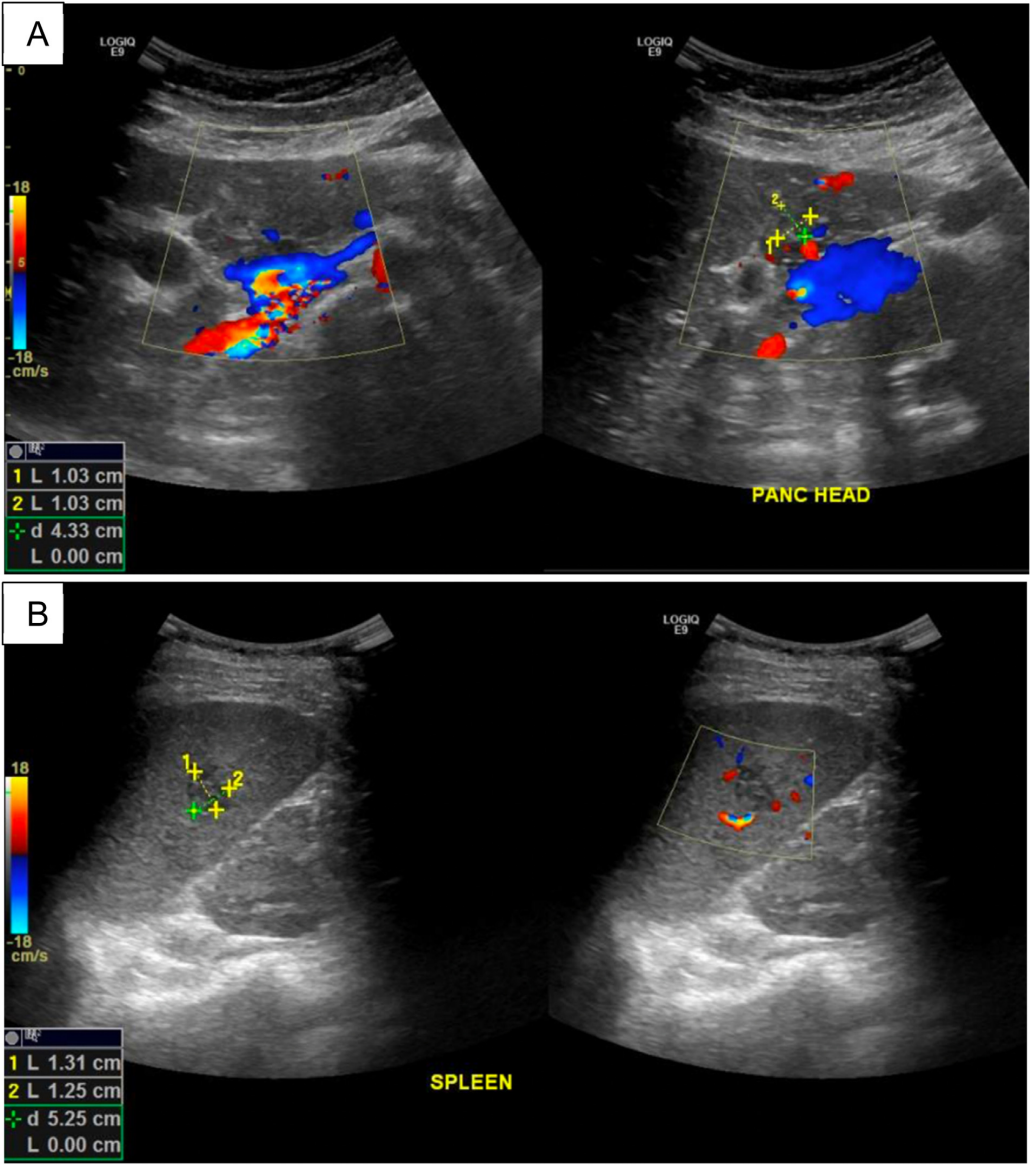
Ultrasound of the abdomen showing ill-defined hypoechogenic lesions in pancreatic head (A) and spleen (B).
